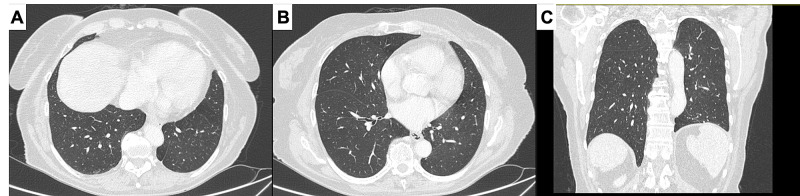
Computed tomography scan of the thorax on axial (A, B) and coronal (C) planes. No ground-glass opacities to suggest novel coronavirus pneumonia nor nodules or secondary lesions involving lung inferior lobes were found.
